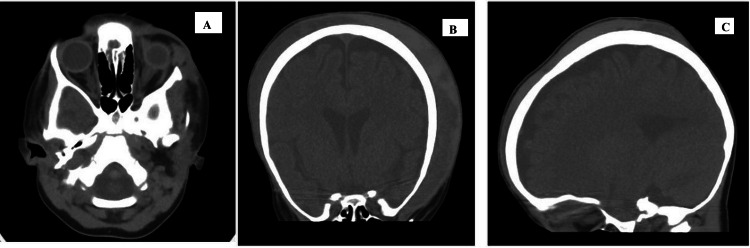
Computed tomography scan of skull and orbits of the child with left orbital hematoma and scalp hematoma. A circumferential scalp hematoma crossing the sutures with evidence of mixed acute and subacute blood density on the left side of the scalp. A left superior orbital roof subperiosteal collection measuring 2.0x0.7x0.7 cm caused proptosis of the left orbit. There was no subarachnoid, intracerebral, or intraventricular hemorrhage.
